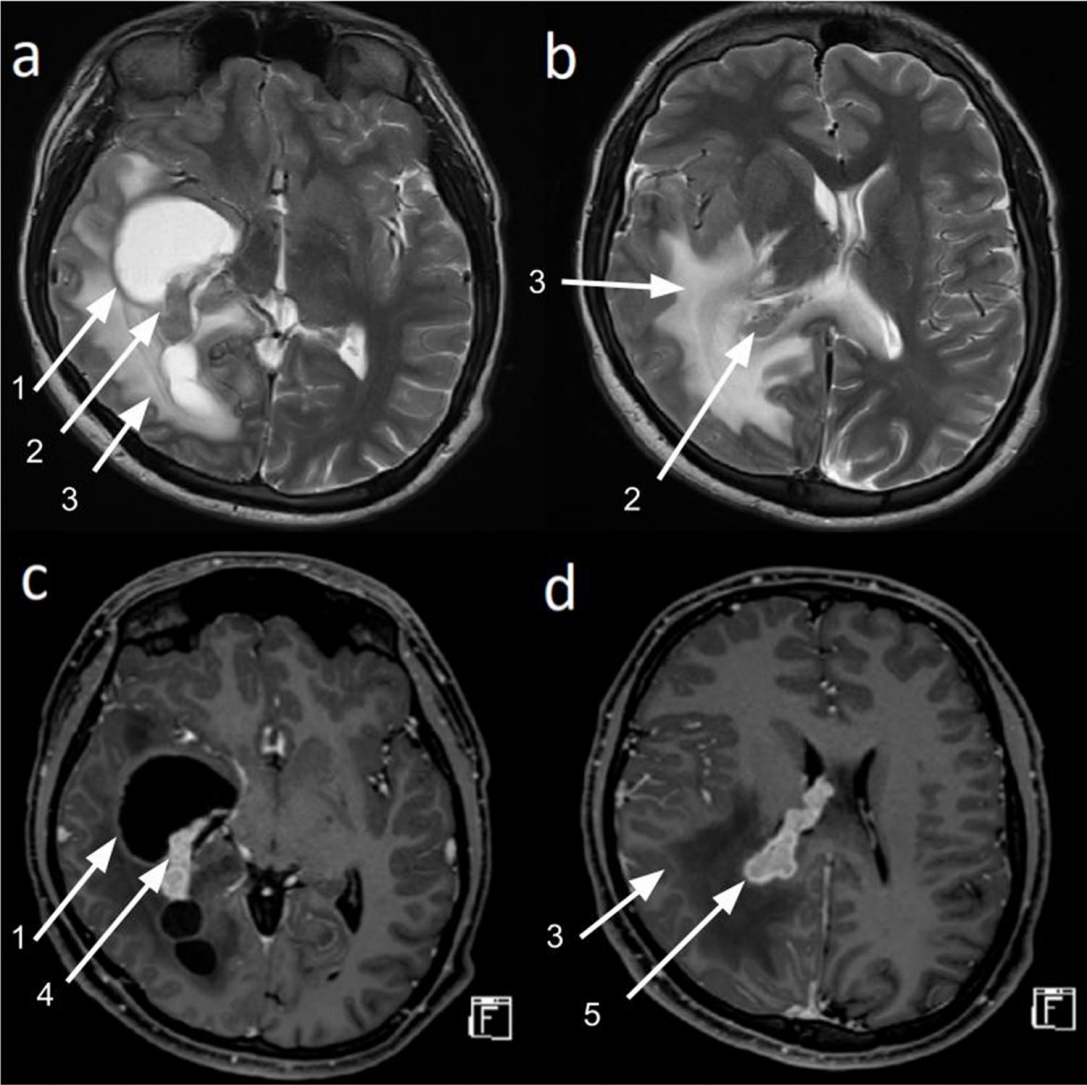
Preoperative magnetic resonance imaging (MRI). a Axial T2: Right-sided trapped temporal horn (1) with intraventricular lesion (2). b Axial T2: Periventricular edema (3)—right temporal and occipital lobe. c Axial T1 + contrast: Contrast-enhancing lesion within the trapped temporal horn (4). d Axial T1 + contrast: Contrast-enhancing lesion arising from the right choroid plexus (5)
